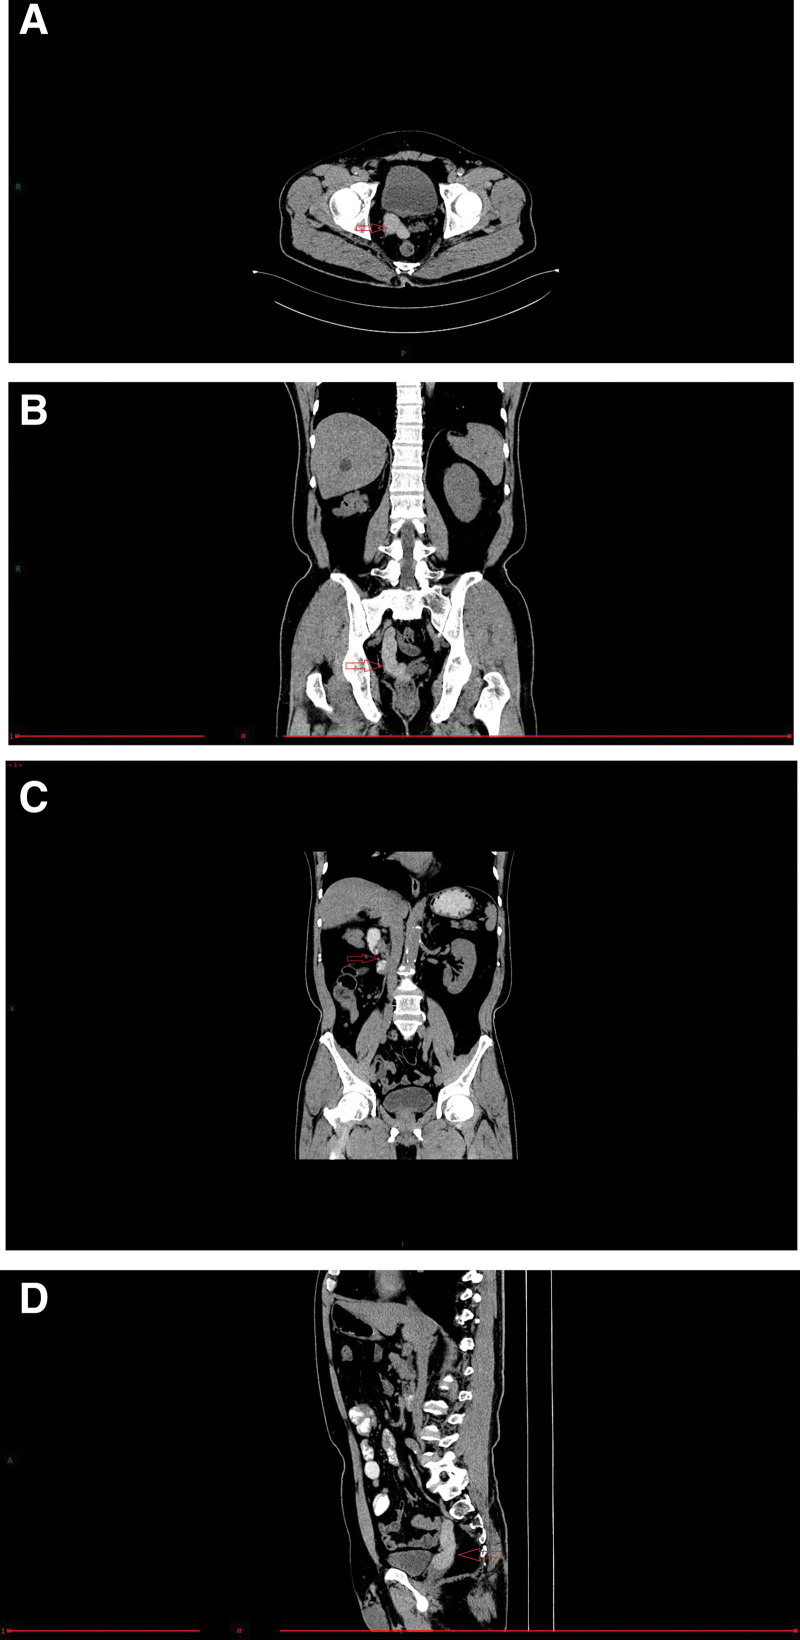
Computed tomography (CT) demonstrates a cord-like mass (arrow) above the right seminal vesicle cyst; (A, B, C) the boundary was clear, with the upper part leading to the “renal region” and the lower part connecting to the right seminal vesicle cyst, and (D) the absence of the right kidney (arrow).
